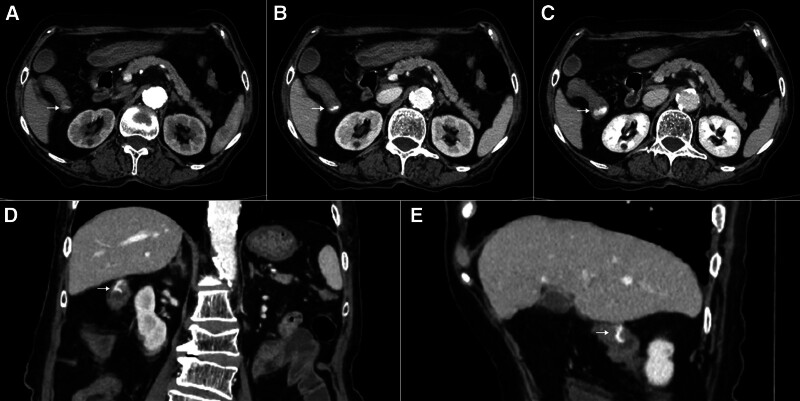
Contrast-enhanced computed tomography manifestations of colonic angiodysplasia. The volume of contrast material within the intestinal lumen increased progressively over time (A–C). The dilated vessels were enhanced within the intestinal wall (D–E). (A) Arterial phase in a horizontal position. (B) Venous phase in a horizontal position. (C) Delayed phase in a horizontal position. (D) Venous phase in a coronal position. (E) Venous phase in a sagittal position.
